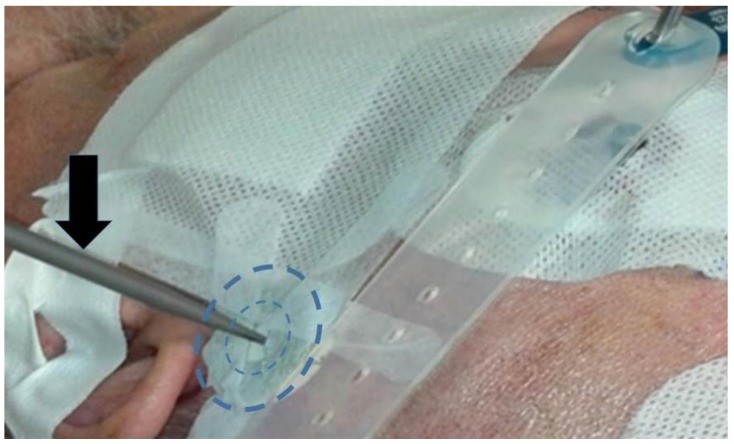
The atraumatic neuro-navigational application of radiopaque circular target fiducials. The navigator probe (in grey) indicates the horizontal entry point (black arrow). The dashed circles represent the profile of X-rays-visible fiducials (see also Figure 3).
